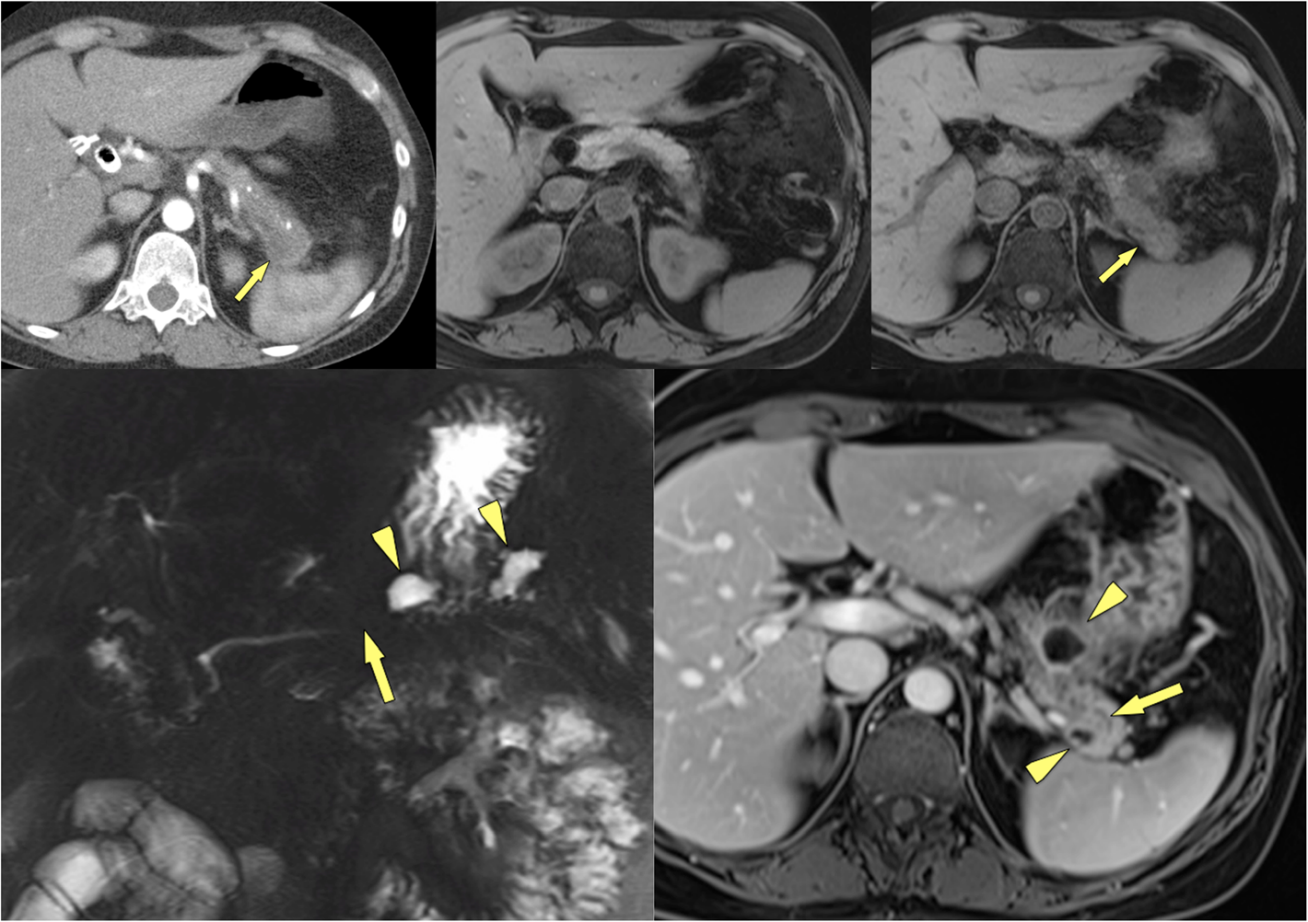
MFCP of the tail (35 y, female). a Incidental note of an pancreatic tail mass (arrow) is made during CT angiography. There are multiple stippled calcification in the tail. b, c T1w GRE fatsat images show normal SI of the body and markedly low SI of the tail (arrow). d MRCP shows a stricture at the junction of pancreatic body and tail (arrow) and two fluid collections in the tail (arrowheads). e Gadolinium-enhanced image shows hypovascular mass and minimal ductal dilatation in the mass (arrow). The two small fluid collections adjacent to the pancreas are pseudocysts (arrowheads). Clues to the diagnosis of MFCP are lack of a duct-obstructing mass at the stricture at the junction of body and tail, presence of pseudocysts and parenchymal calcifications (at CT)
